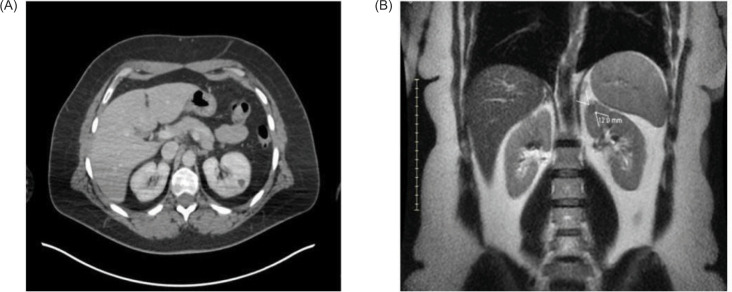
Small renal mass in the upper pole of the left kidney as seen on CT and MRI. (A) Axial view from CT demonstrates a 12 mm lesion. (B) Coronal view from the abdominal MRI also shows a solitary 12 mm upper pole anterior endophytic lesion of the left kidney. Pathology indicates metastatic ocular melanoma.
